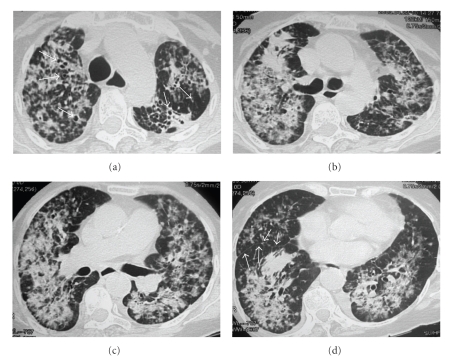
HRCT scans at four levels (a)–(d) demonstrate confluent airspace nodules and areas of consolidation in both lungs, with signs of architectural distortion that are characterized by bronchial dilatations within the lesions (white arrowheads in (a)) and fissure distortion with loss of the regularity (white small arrows in (d)).
